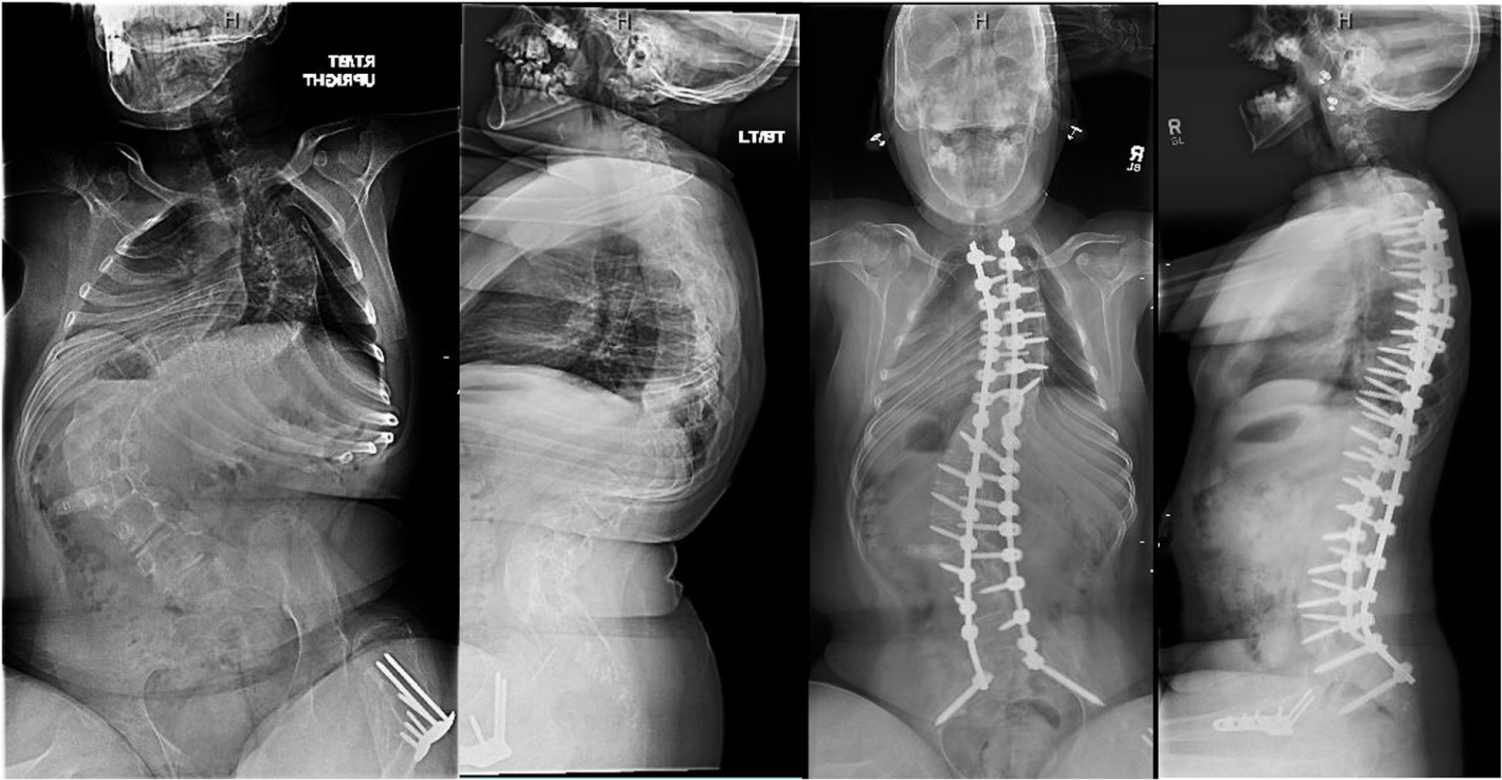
Pre- and 1-year postoperative anteroposterior and lateral radiographs of a 13-year-old female with spastic quadriplegic cerebral palsy treated by posterior spinal fusion from T2 to pelvis showing satisfactory correction. Intraoperative blood loss was 400 mL and perioperative decrease in Hb was 2.3 mg/dL
